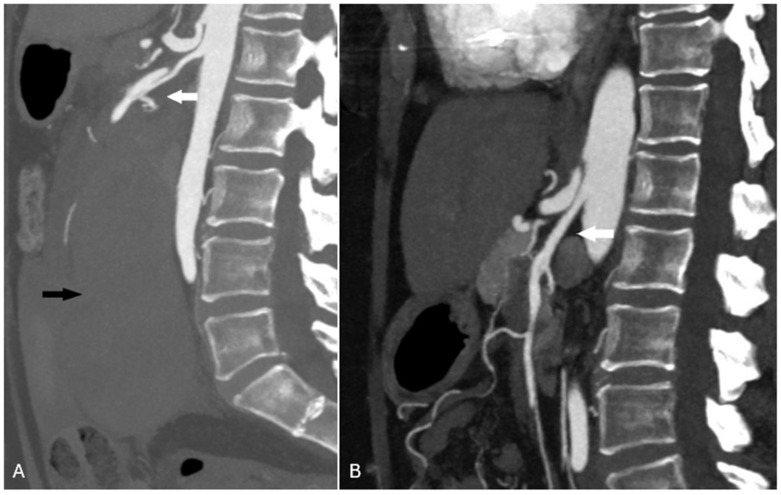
(A) Sagittal reformatted CT angiogram showing acute dissecting aneurysm of the proximal superior mesenteric artery (white arrow) and large 14 cm × 13 cm mesenteric hematoma with associated hemoperitoneum (black arrow). (B) 18-month follow-up saggital reformatted CT angiogram demonstrating healed superior mesenteric artery (white arrow).
